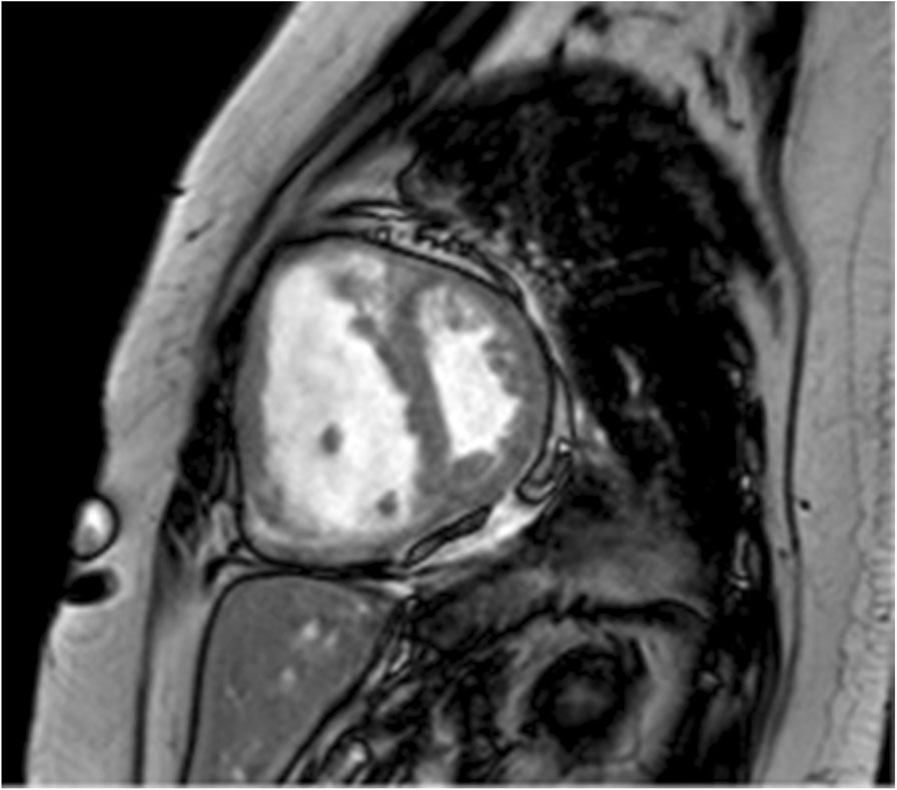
Pulmonary arterial hypertension. Short axis balanced steady state free precession cine image in patient with precapillary pulmonary hypertension in diastole demonstrating right ventricular dilatation and hypertrophy as well as bulging of the interventricular septum into the small left ventricle as a sign of high pulmonary arterial pressure. The septal flattening can easily be demonstrated by echocardiography, but the biventricular mass, volumetric, and functional quantification using CMR is superior to echocardiographic estimates
